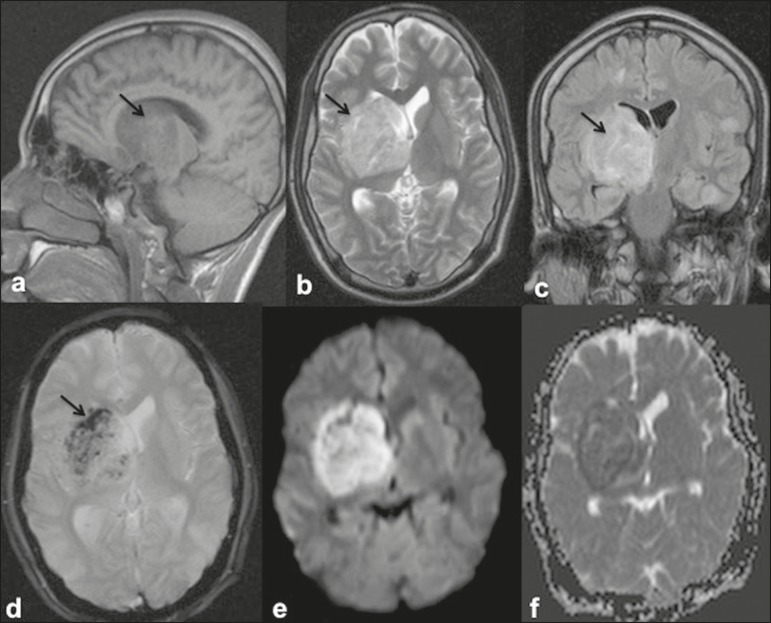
16-year-old female with dengue encephalitis. Sagittal T1-weighted image
